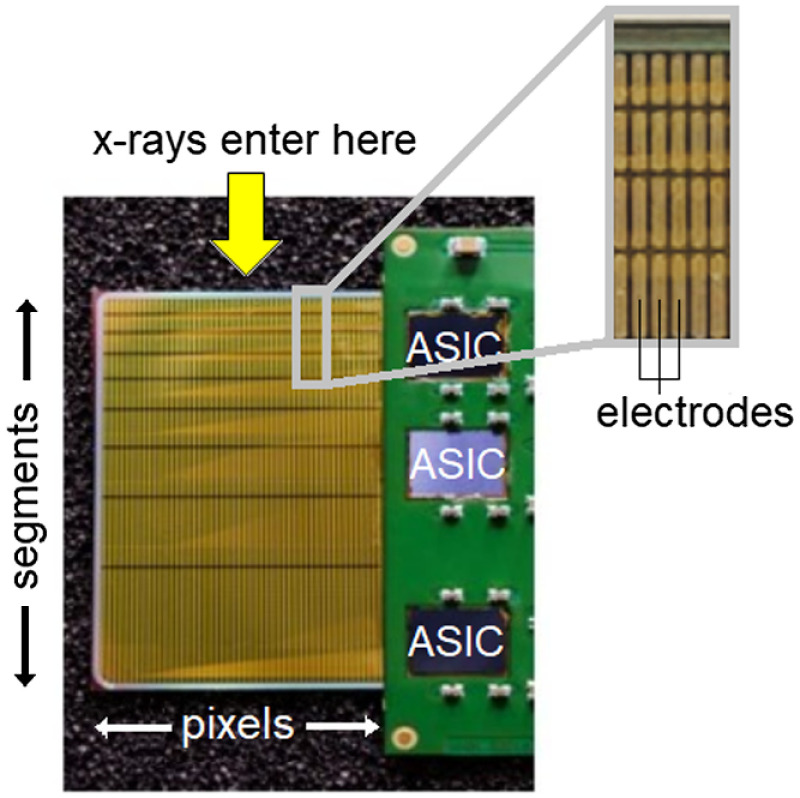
Photograph of a segmented silicon strip detector module. The module is oriented with its upper edge toward the x-ray source. Three wire-bonded ASICs are visible as the black rectangular shapes to the right.
